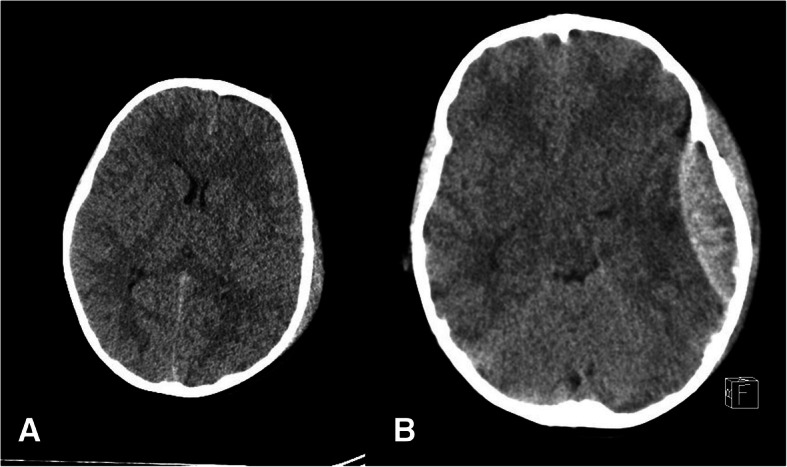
CT scan at admission (a) and 3 h after admission (b) of children presenting with early clinical deterioration and a typical lucidity interval
